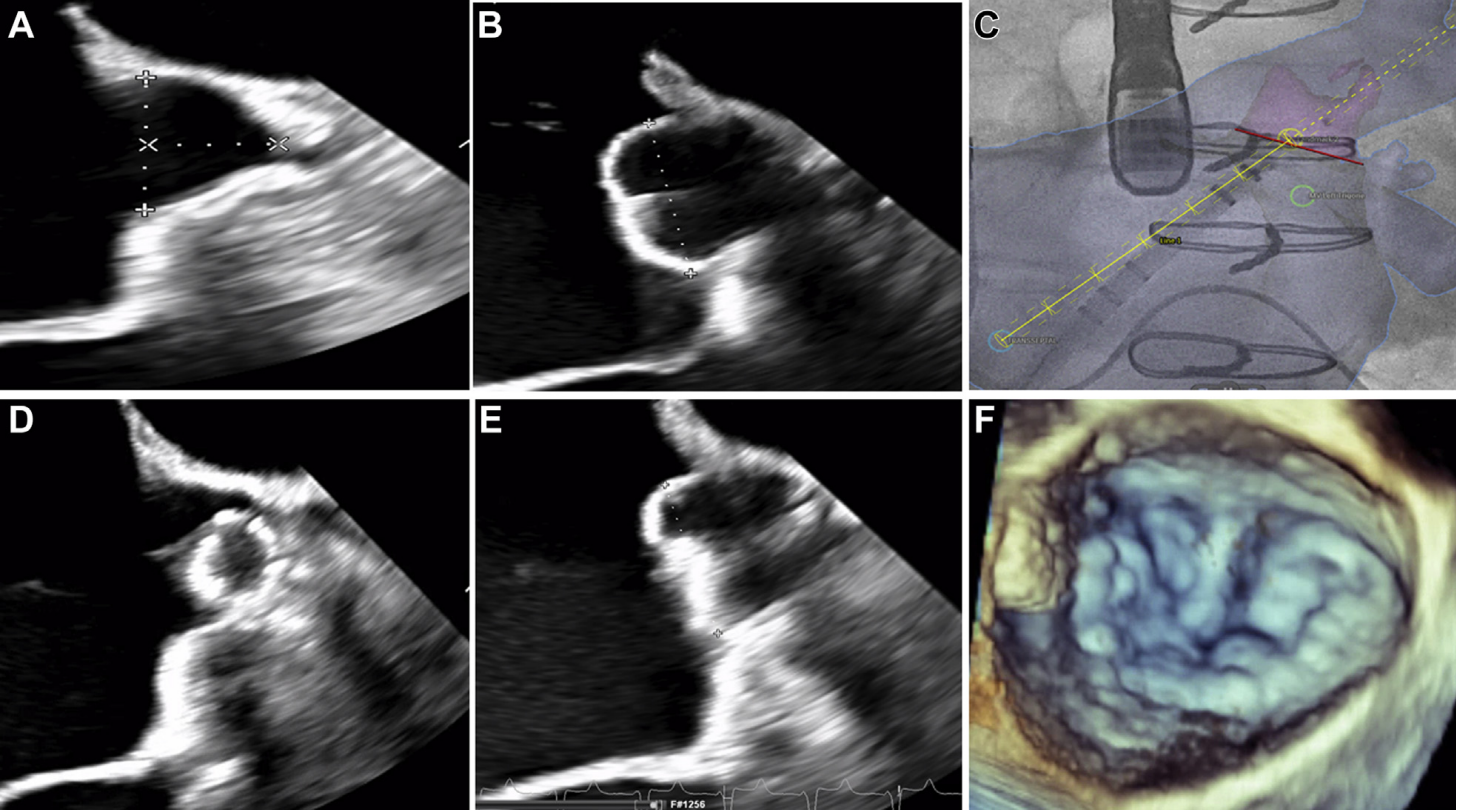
Watchman FLX Implantation (A) Left atrial appendage (LAA) stump at 45°. (B) Inadequately seated 24-mm new generation LAA closure device. (C) Use of fluoroscopy with CT overlay to place 20-mm new generation LAA closure device. (D) The device in a Flex ball configuration positioned distally. (E) A well-seated device with adequate compression. (F) 3-dimensional transesophageal echocardiogram image of the device covering LAA ostium.
